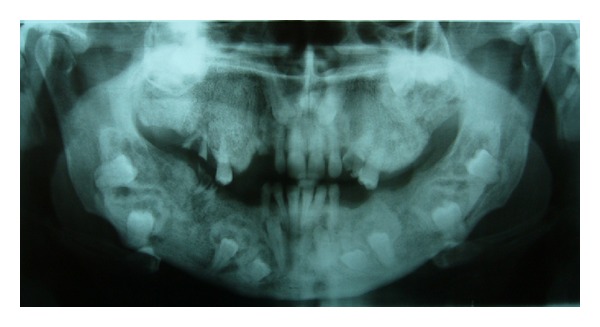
Preoperative panoramic radiograph showing multiple impacted teeth and bone expansion.
